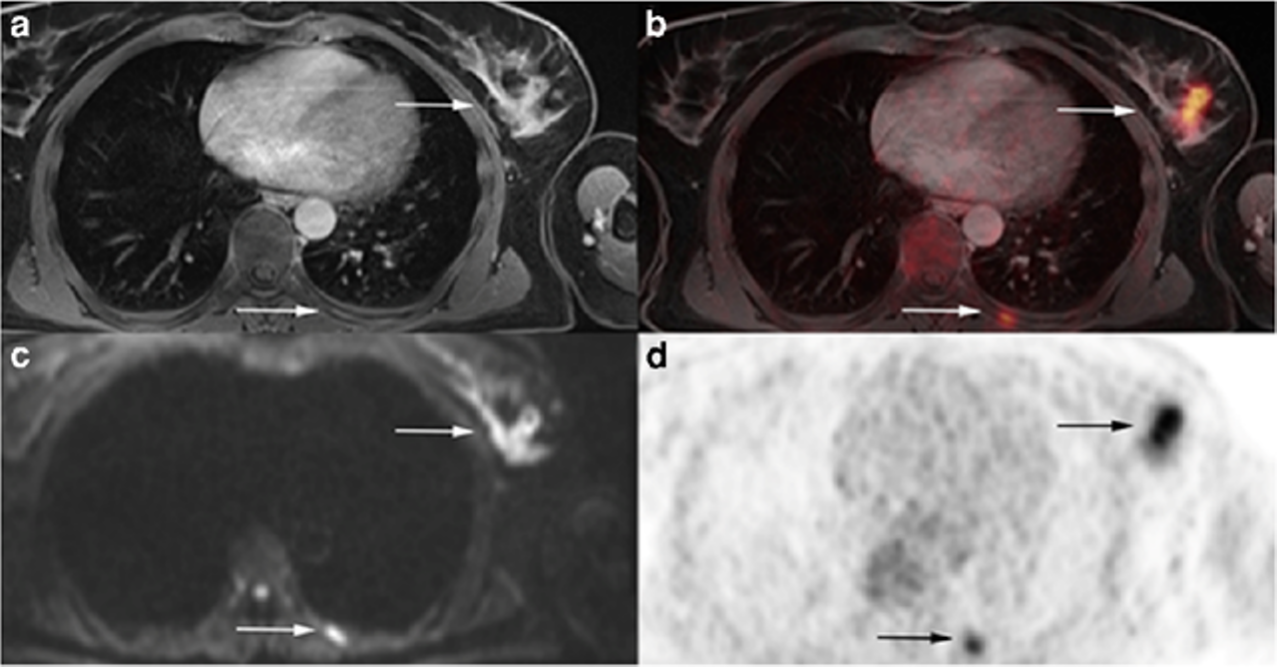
A 57-year old woman with diagnosis of primary breast cancer. Primary tumor located in the left breast and visible bone metastasis in a left rib with contrast enhancement on T1w fs VIBE (a), corresponding diffusion restriction (c), and pathological FDG uptake on PET (d) and fused ^18^F-FDG PET/MRI (b)
